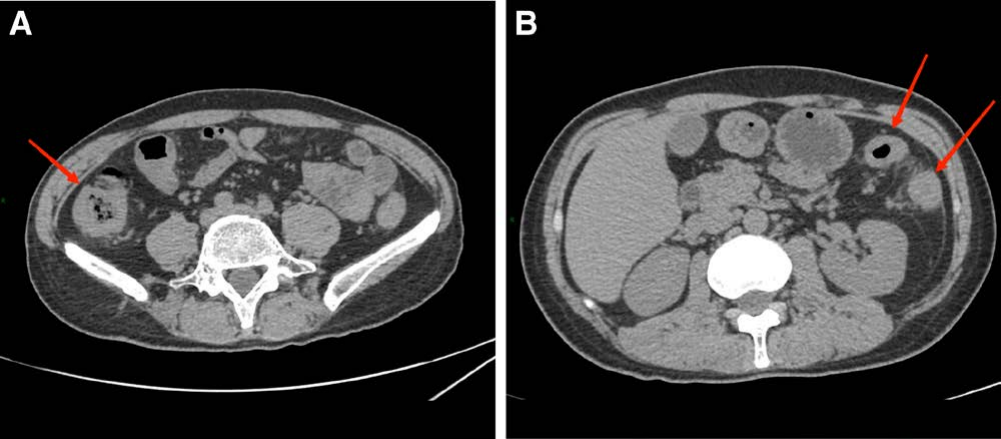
CT image of the abdomen: Images show thickening of the walls and surrounding infiltration in the cecum ascending colon, transverse colon, and descending colon(arrows), suggestive of inflammatory lesions.
